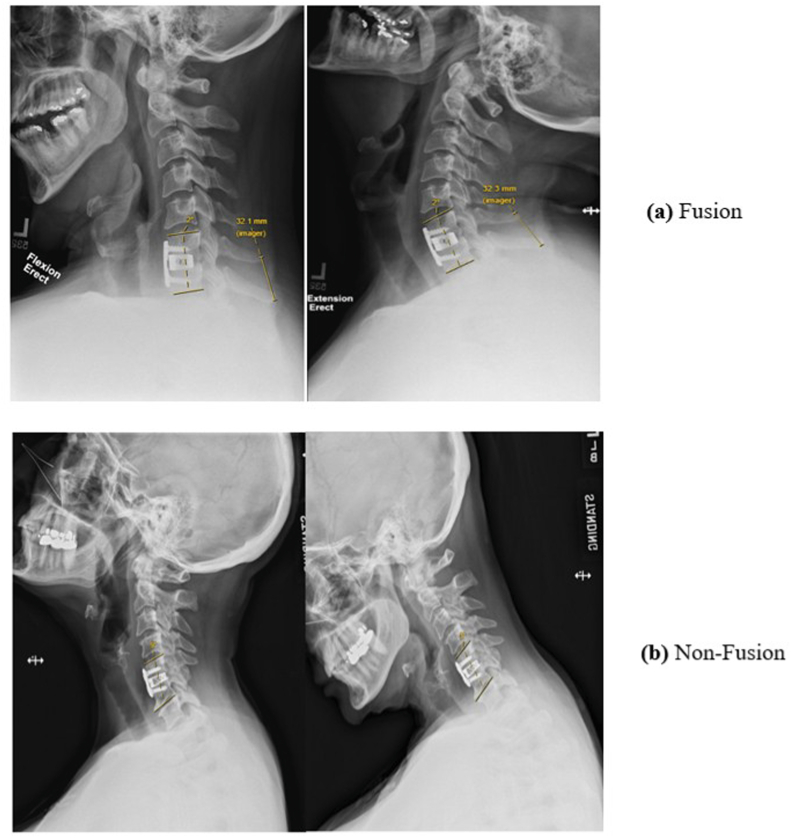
Demonstration of Fusion Assessment: (a) Fusion target level demonstrated less than 3.0° difference in Cobb angle and less than 2.0 mm difference in interspinous distance comparing flexion and extension radiographs. (b) the two required conditions were not fulfilled.
